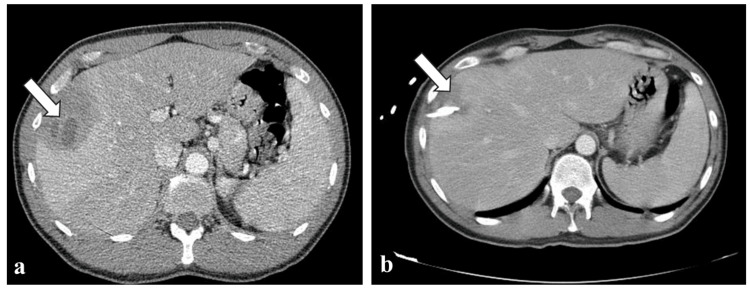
CT scan of the upper abdomen. CT scan of the upper abdomen revealing a septated non-homogeneous right hepatic lobe mass (arrow), compatible with a chronic abscess, with “cluster and double target sign”, defined as a thick walled multiloculated lesion (cluster sign) with central low attenuation fluid-filled component surrounded by a high attenuation inner rim and a low attenuation outer ring (double target sign) before (a) and after (b) CT-guided insertion of catheter and drainage.
